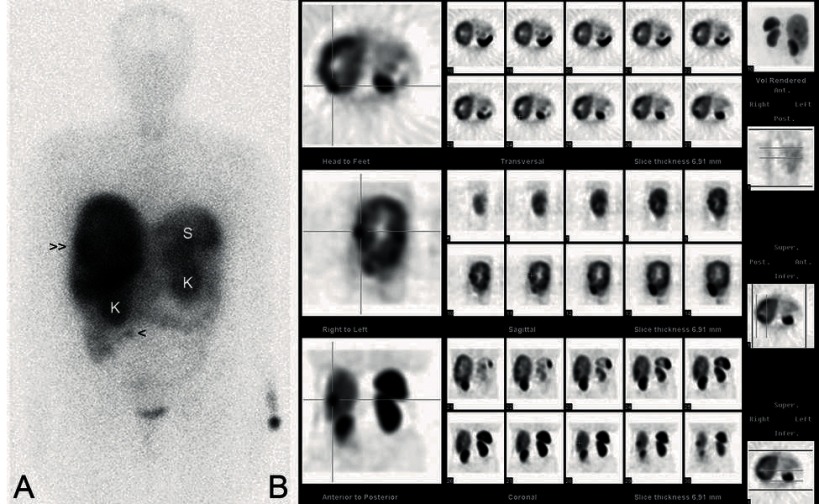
Whole-Body Scintigraphy and Single Photon Emission Computed Tomography (SPECT). Whole-body scintigraphy anterior view (A) and single photon emission computed tomography (SPECT) in the transversal, sagittal and coronal planes (B) after intravenous injection of indium-111-octreotide, showing a large hyperenhanced area that occupies almost the entire right hepatic lobe (double arrowhead) with several focal accumulations of high intensity in both lobes, compatible with an hepatic infiltration by a tumor expressing somatostatin receptors. Also, a focal uptake distal to the inferior pole of the right kidney and apparently localized in the small intestine is seen (arrowhead). Both kidneys uptake the Indium-111-octreotide because it is cleared from the body primarily by renal excretion. Hepatobiliary excretion represents a minor route of elimination, and less than 2% of the injected dose is recovered in feces within three days after injection. Also, ten percent of the radioactivity is excreted as a nonpeptide-bound. Normal distribution of Indium-111-octreotide can be found in the pituitary gland, thyroid gland, liver, spleen, kidneys, urinary bladder and the bowel. L: liver, K: kidney, S: spleen.
